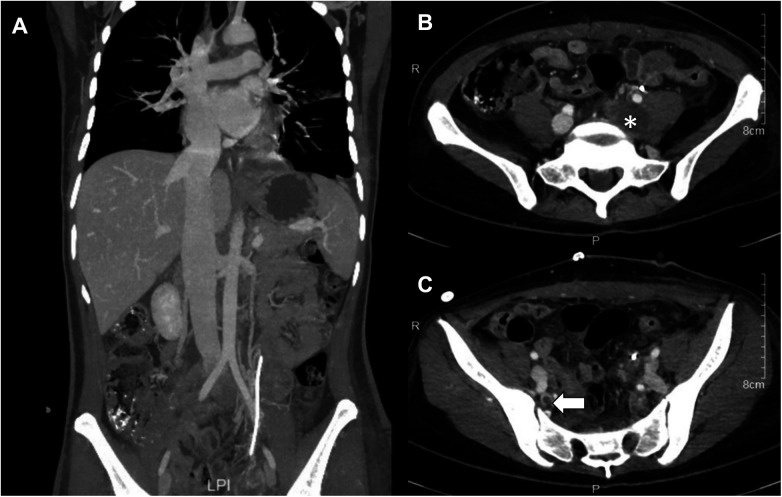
Postoperative computed tomography venography (CTV) at 6-day follow-up. (A) Coronal view demonstrating the complete resolution of the tumor thrombus in the right atrium, right ventricle, left ovarian vein, and inferior vena cava. (B) Axial view showing residual tumor thrombus in the left common iliac vein (asterisk). (C) Axial view showing residual tumor thrombus in the right internal iliac vein (arrow).
